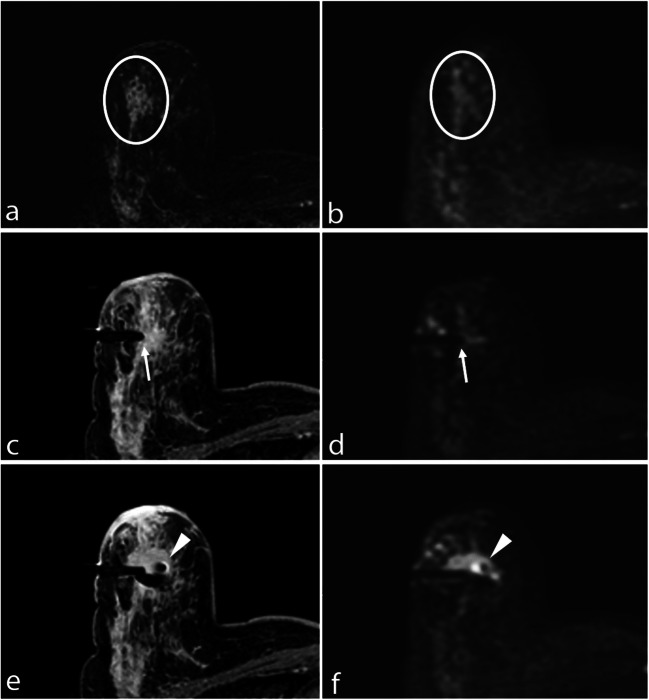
a Subtracted DCE 3D-THRIVE showing a non-mass lesion in the upper outer quadrant of the right breast of a 51-year-old woman (circle). b DWI (*b* = 1300 s/mm^2^) showing hyperintensity of the enhancing lesion (circle). c T1-WI FS GRE showing the proper position of the biopsy device (arrow); the needle is well recognizable at (d) DWI (*b* = 1300 s/mm^2^, arrow). e T1-WI FS GRE showing the correct deployment of the site marker that is also recognizable at (f) final DWI (*b* = 1300 s/mm^2^, arrowhead)
